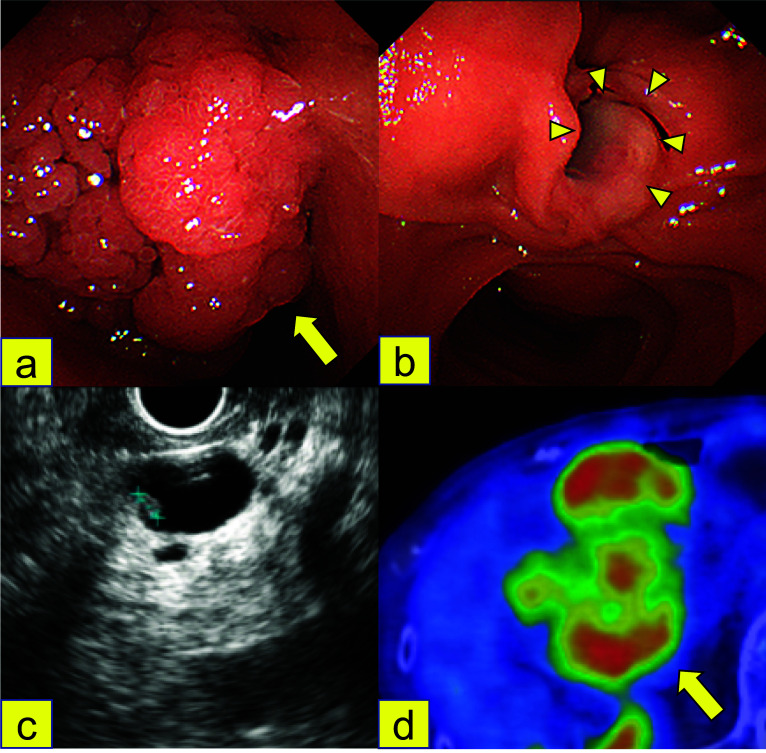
Examination and imaging findings. a EGD showed a semi-circumferential papillary tumor protruding toward the duodenal lumen (arrow). b The mucosa of the Vater papilla was intact (arrowhead). c EUS revealed a flat isoechoic mural nodule 4 mm in diameter in the dilated main pancreatic duct of the pancreatic head. d FDG-PET-CT showed an accumulation of SUV max 6.75 in the pancreatic head tumor (arrow)
